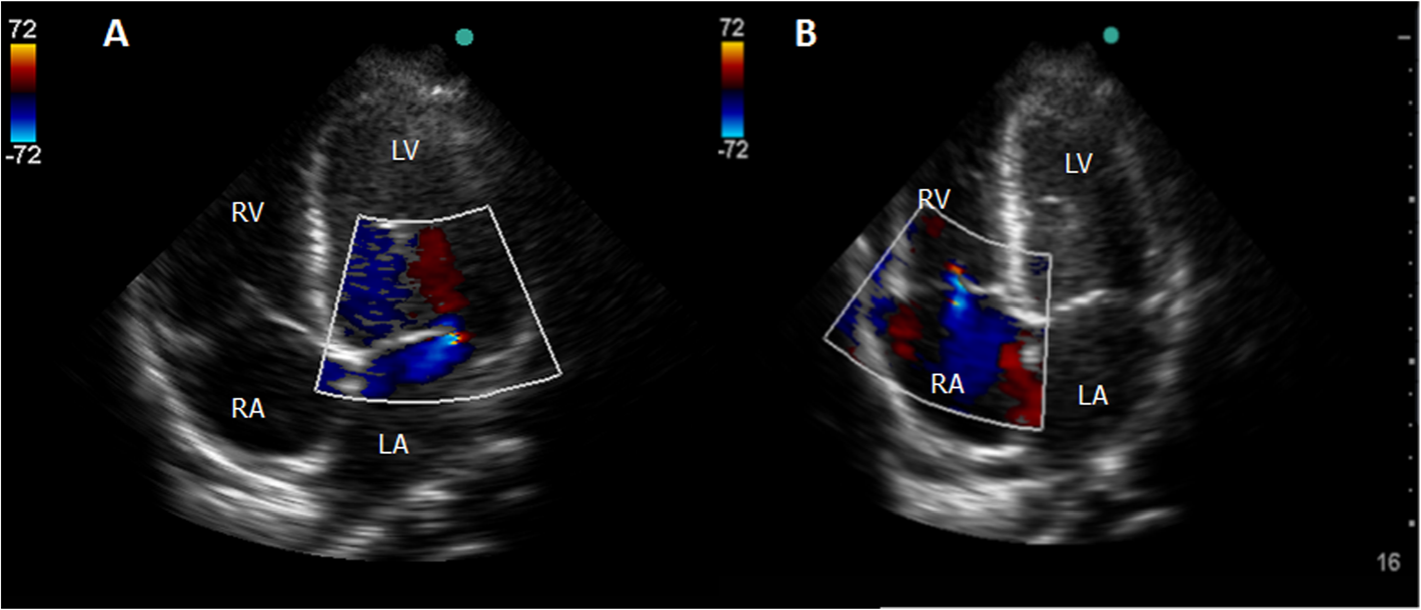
Valvular regurgitation. A- Mild MR by color Doppler (apical 4-chamber view). B- Moderate TR by color Doppler (apical 4-chamber view). LA, left atrium; LV, left ventricle; RA, right atrium; RV, right ventricle.
